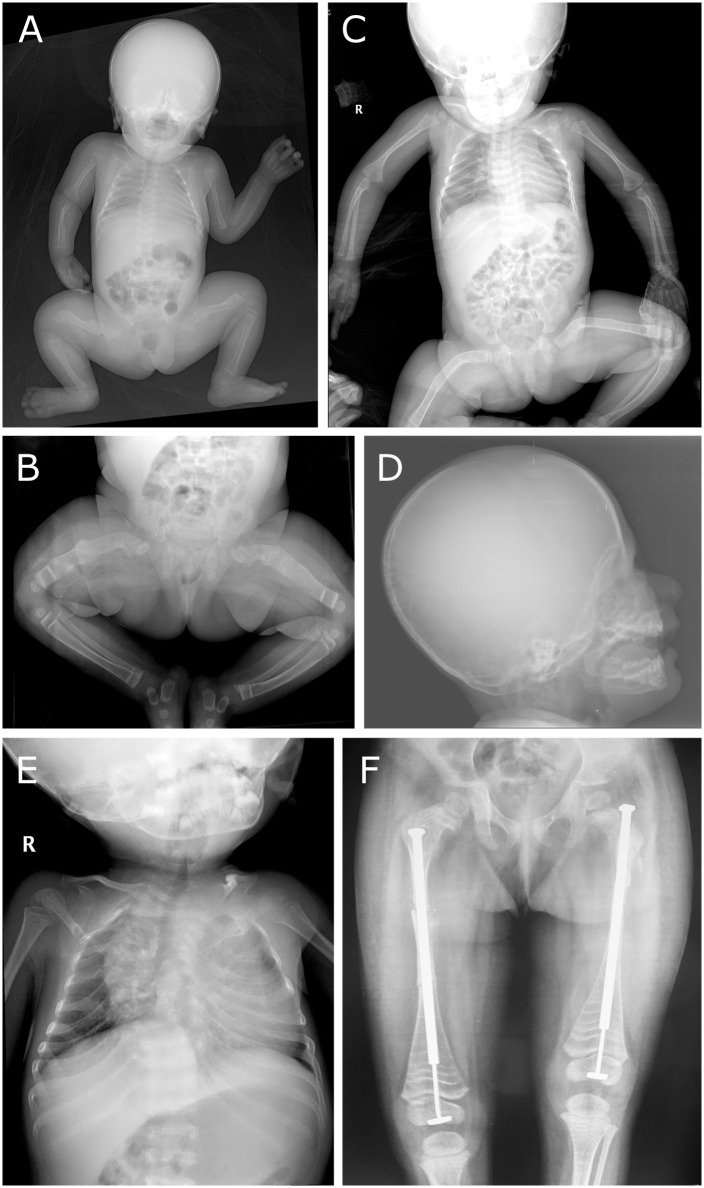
Radiological features. (A-C) X-rays at age 4 days (A), 2 months (B) and 8 months (C) show fractures with callus formation, gracile and thin ribs with fractures, platyspondyly and severe hypomineralization. (D) Skull X-ray at age 10 months shows severe thinning and Wormian bones. (E-F) X-rays at 3 years of age show crowding of the ribs, scoliosis and femora with ‘Zebra lines’ following regular pamidronate infusions.
